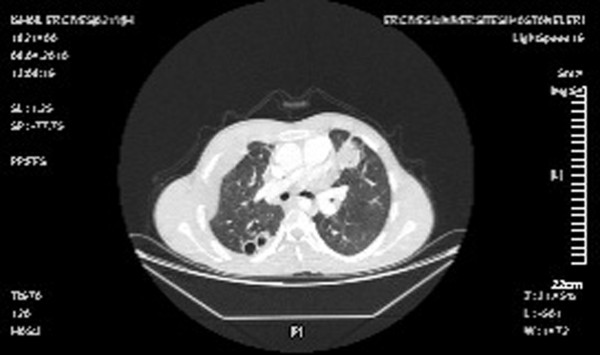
Computed tomographic scan of a 20-year-old male demonstrates bilateral multiple and perforated lung cysts four months after medical treatment.
